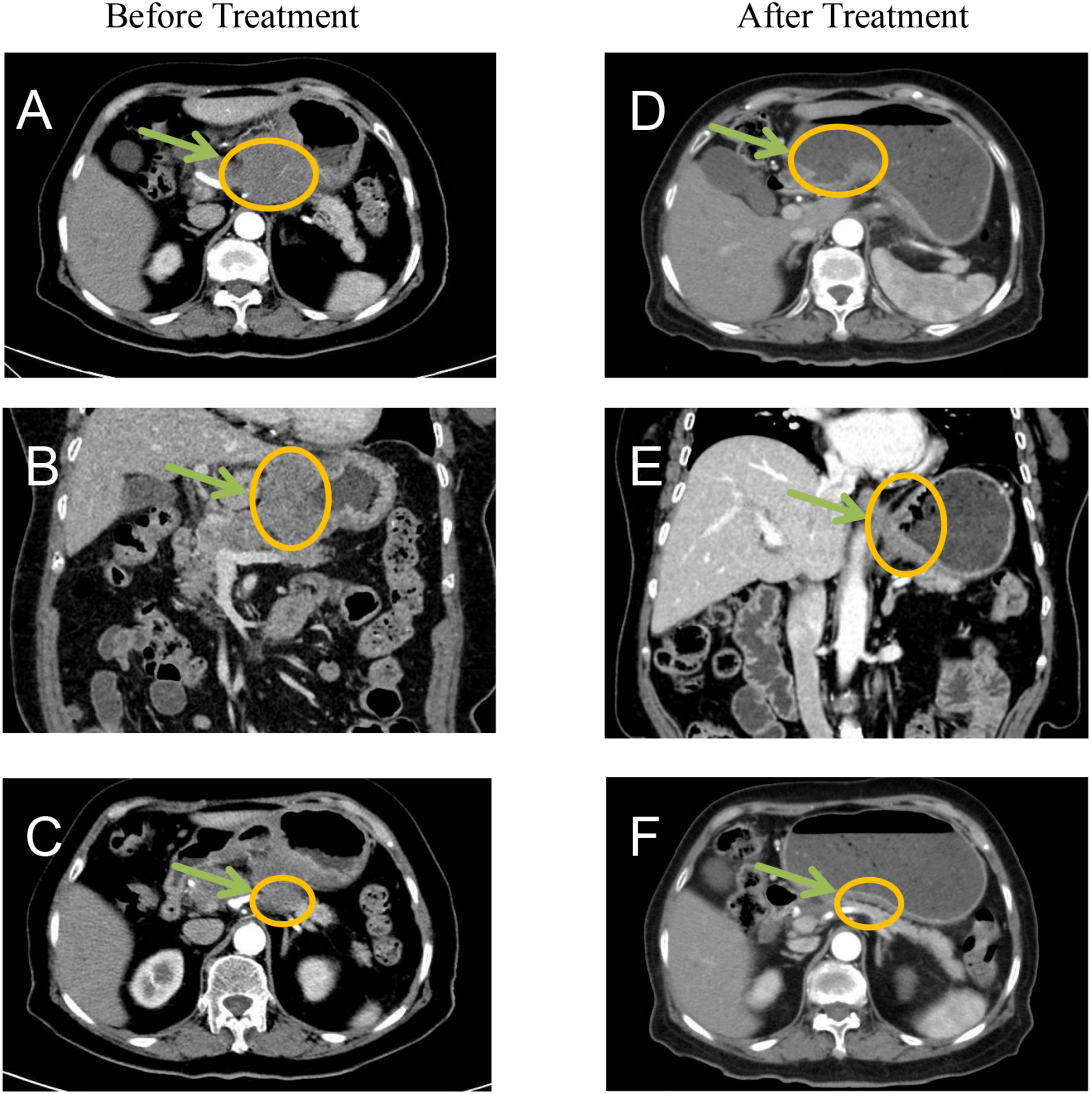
Before treatment CT imaging demonstrated: (A, B) Marked thickening and nodularity along the gastric curvature with contrast enhancement, showing poorly defined margins between the stomach and pancreas. (C) Significant enlargement of lesser curvature lymph nodes. After treatment imaging revealed: (D-F) Substantial reduction in both the primary tumor mass and associated lymphadenopathy, indicating favorable treatment response.
